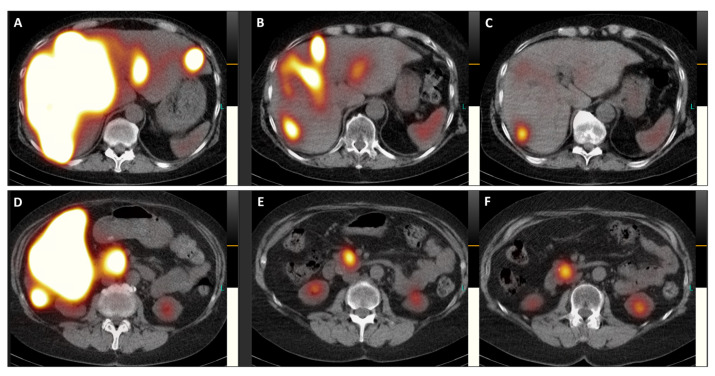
Transversal images of the post-therapeutic SPET/CT scans (Figure 5, (A,D), July 2023; (B,E), September 2023; (C,F), November 2023) provide a detailed view of the remarkable reduction in SSTR antagonist uptake in both liver lobes and the pancreatic head, accompanied by a decrease in the size of the primary tumor (from 41 × 41 mm in July 2023 to 34 × 34 mm in November 2023).
